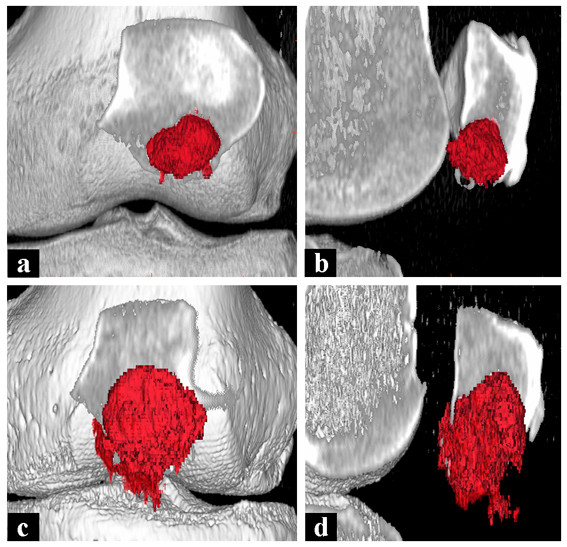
CT-scans with three-dimensional reconstruction and volume analysis. Three-dimensional reconstructions of CT-scans showing the significant progress of the lesion from May (a + b) to August 2008 (b + c).
